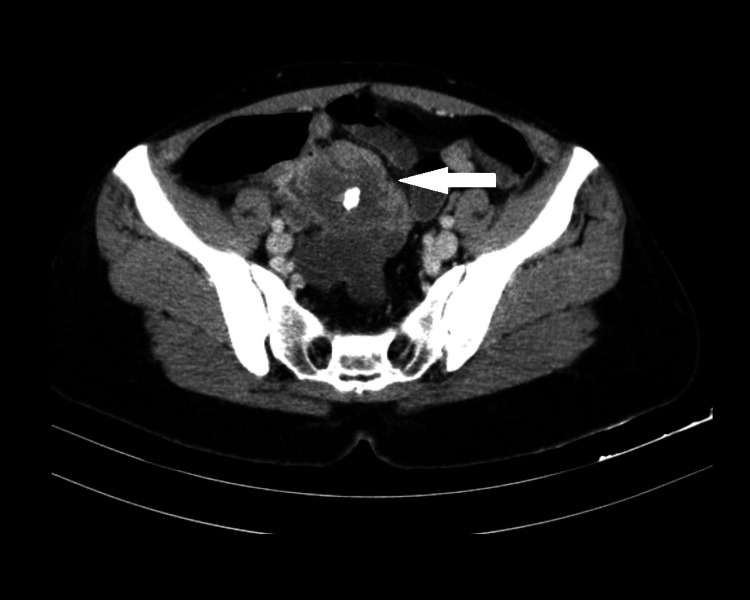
Abdominal-pelvic CT scan
